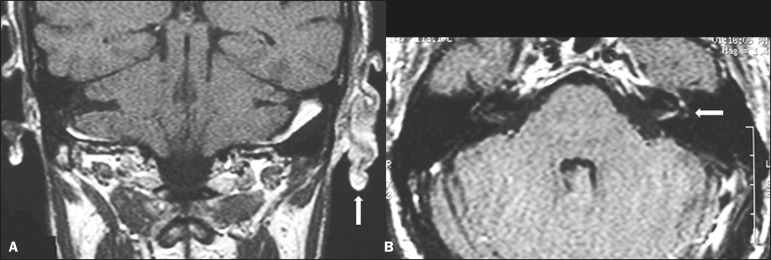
Ramsay Hunt syndrome. A: Coronal T1-weighted MRI sequence showing
